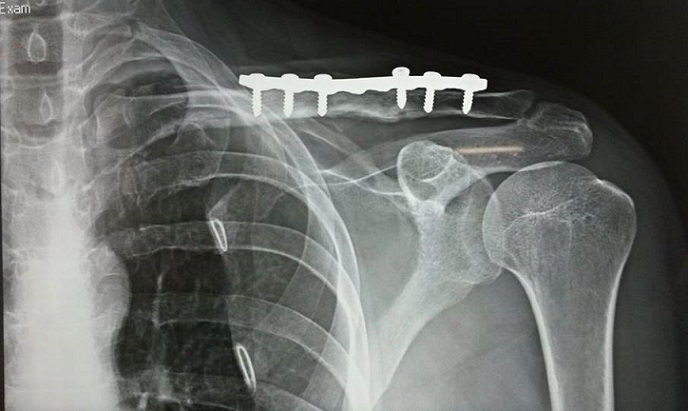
Chest radiographs showing callus formation of the fractured clavicle 14 months after the injury with no recurrence of pneumothorax
